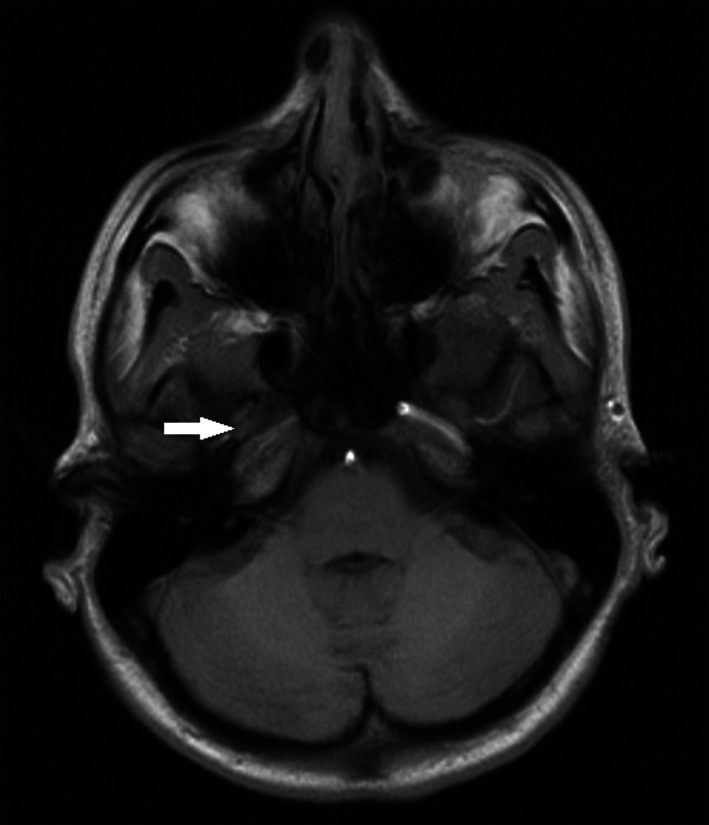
Case 2: occlusion of the right internal carotid and middle cerebral artery marked with an *arrow* (MRI)
